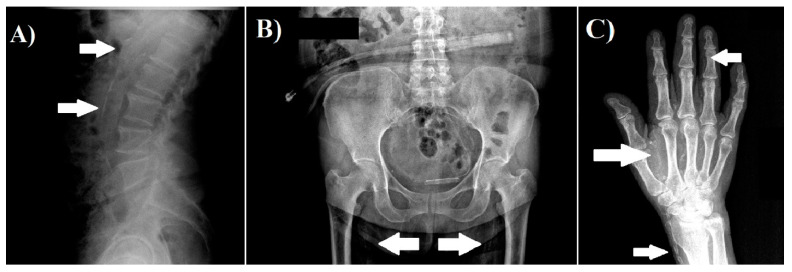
Examples of vascular calcification. (A) Lateral abdomen radiograph, which shows the presence of vascular calcification in aorta. (B) Pelvis radiograph, which shows the presence of vascular calcification in the iliac arteries. (C) Hand radiograph, which shows vascular calcification in the radial, palmar arch, and digital arteries.
